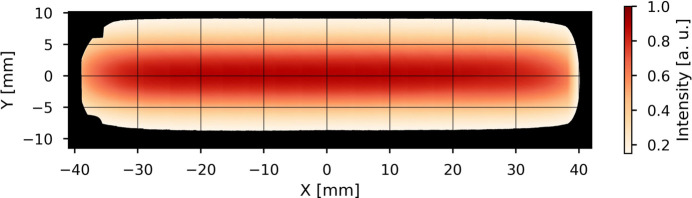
Profile of the filtered white beam at the sample position (43 m from the X-ray photon source) inside the BEATS experimental station. The beam was filtered with 5 mm of glassy carbon and 5 mm of silicon, for a resulting peak X-ray energy of 36 keV. The image is a mosaic of 20 flat-field images collected with 13 µm pixel size and no sample in the detector field of view. When all slits are removed from the beam path, the detected beam edges are shaped by the oval geometry of the copper frame holding the second CVD diamond window. A usable beam size of 75 mm horizontally and 15 mm vertically is available for experiments.
